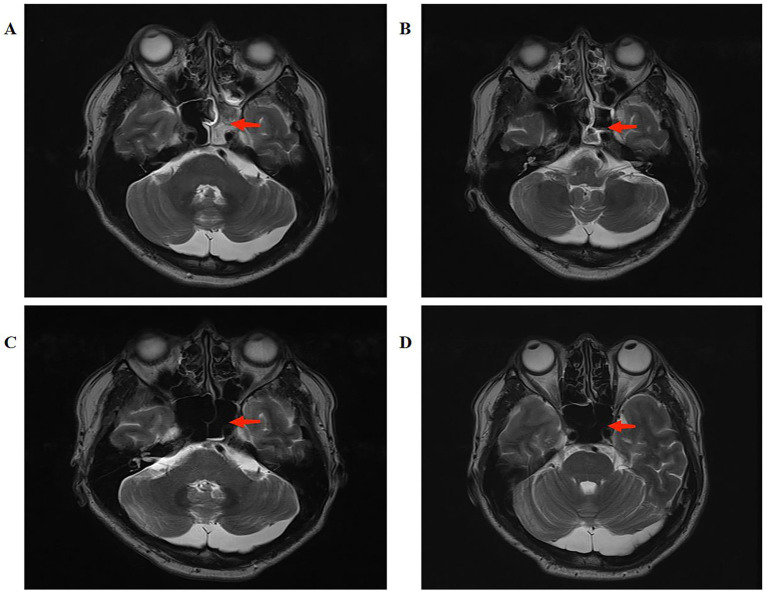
Contrast-enhanced MRI demonstrating cavernous sinus thrombosis at presentation (A); follow-up MRI at 1 month (B); at 3 months (C); and at 8 months (D), showing progressive resolution.
